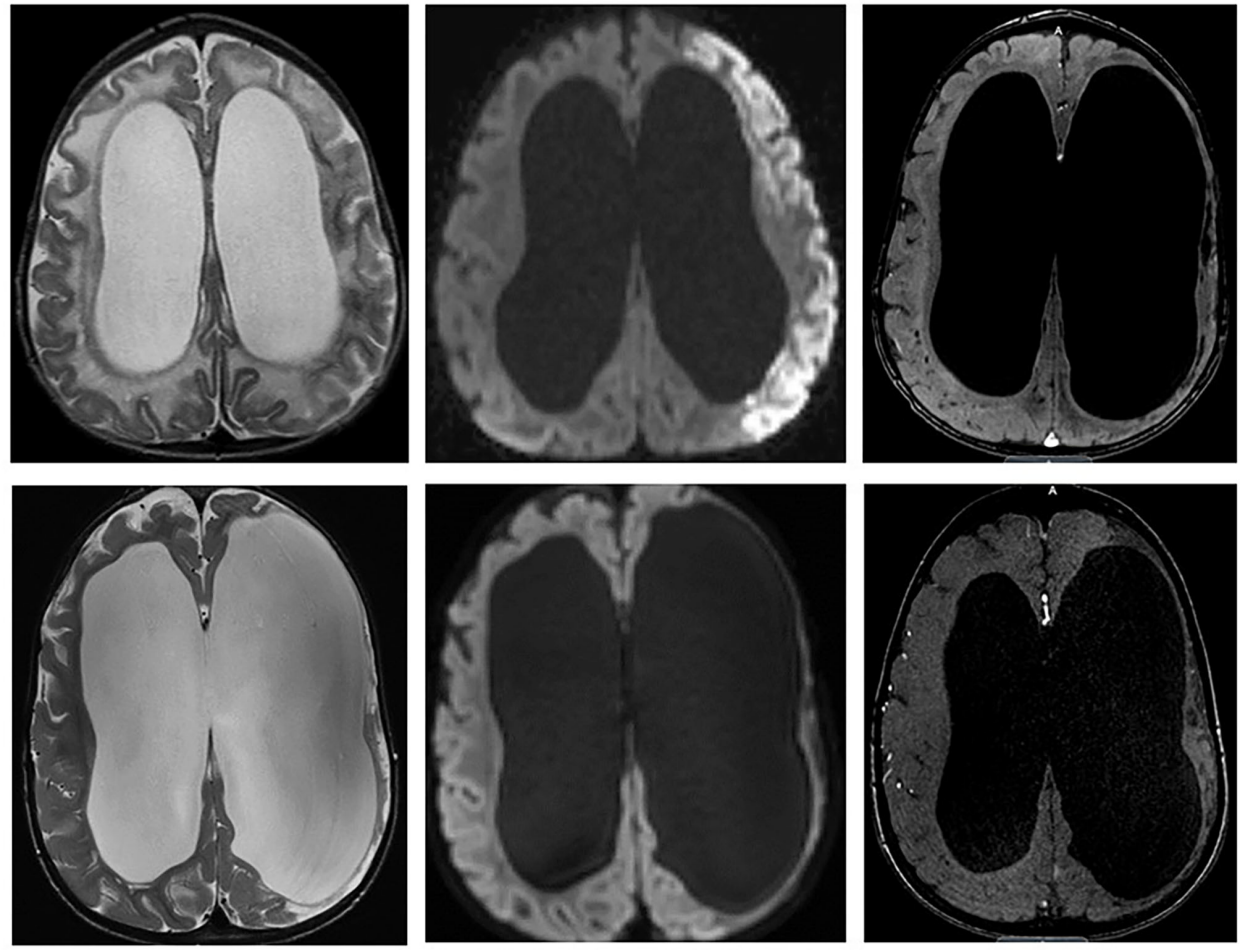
(Upper) Initial MRI of left MCA overt stroke identified on T2, diffusion, and MRA. (Lower) Two years after right cerebral revascularization demonstrating no new ischemic or infarcted territories and increased right cerebral cortical vascularity.
